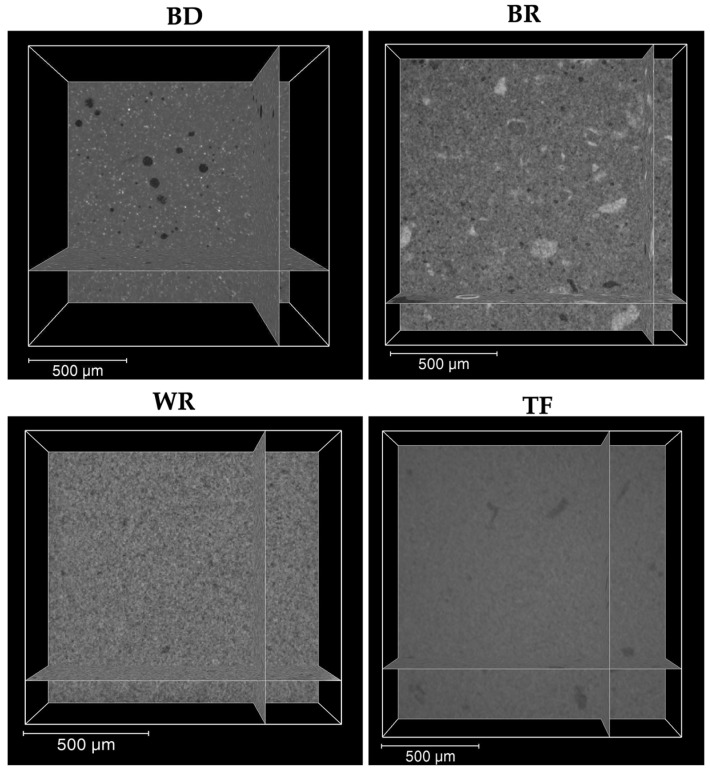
Micro-computed-X-ray-tomography 2D slices showing the internal structure of the three cements (Biodentine “BD”, MTA Biorep “BR” and Well-Root PT “WR”) and Total Fill sealer ‘’TF’’.
